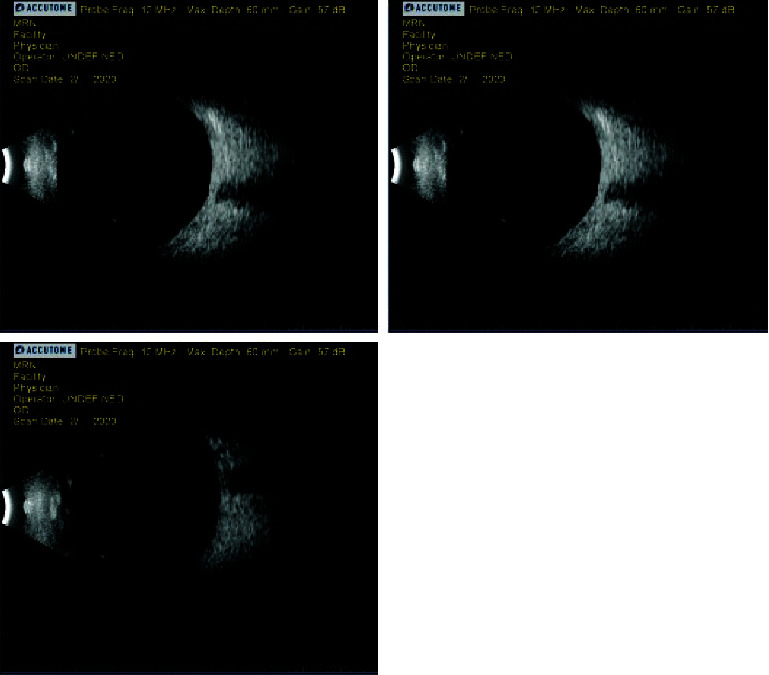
Ultrasound B-scan of the left eye on postop day 7. No choroidal detachment or hemorrhage.
